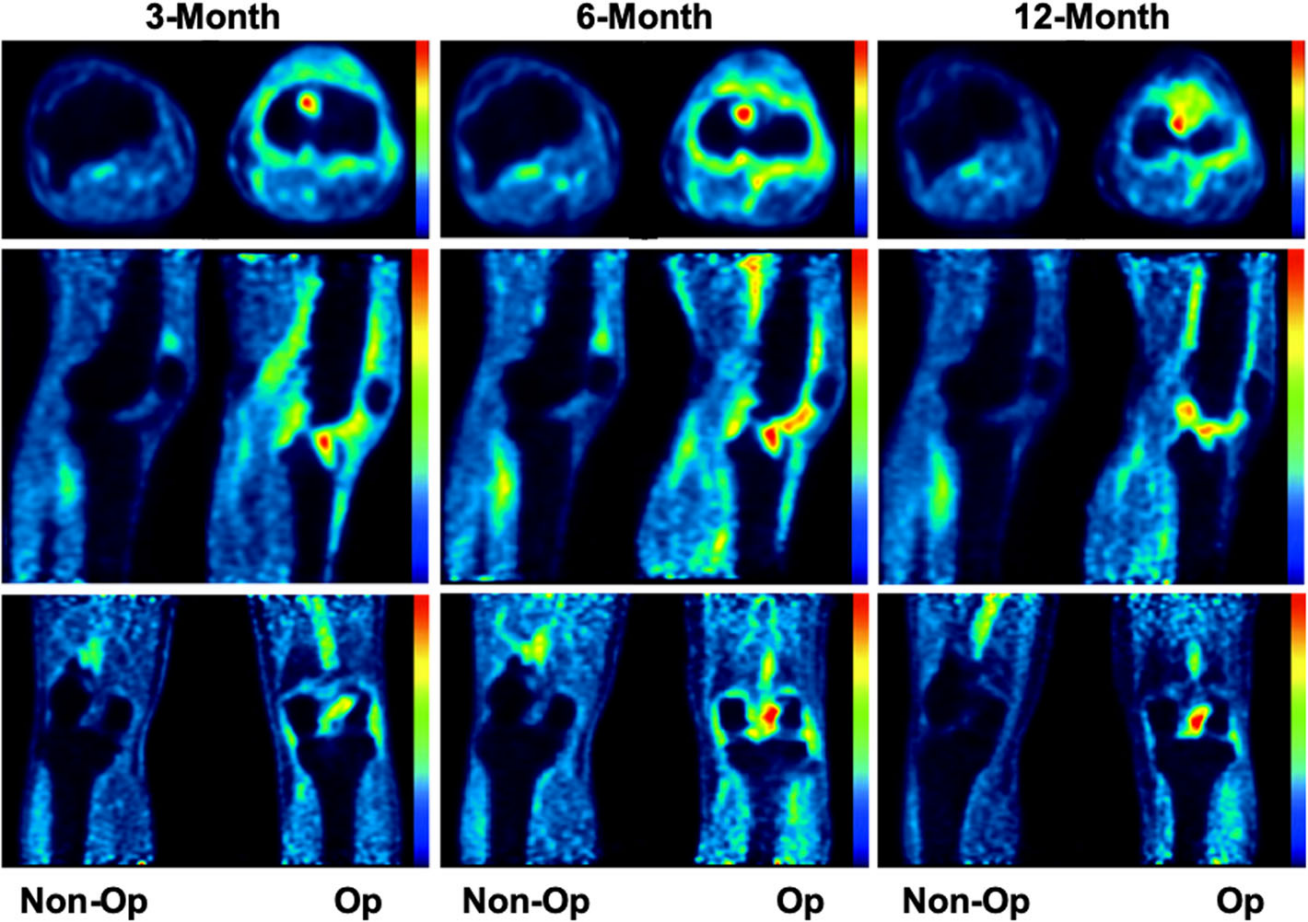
Time course of PET uptake following ACL reconstruction. Whole knee PET uptake regions of interest in non-operative (left) and operative (right) knees 3 months, 6 months, and 12 months post-operatively from a single representative patient. Images are taken from axial (top), sagittal (middle), and coronal (bottom) planes centered on the ACL. Increased ^18^F-FDG uptake is indicated by a shift from blue to red
